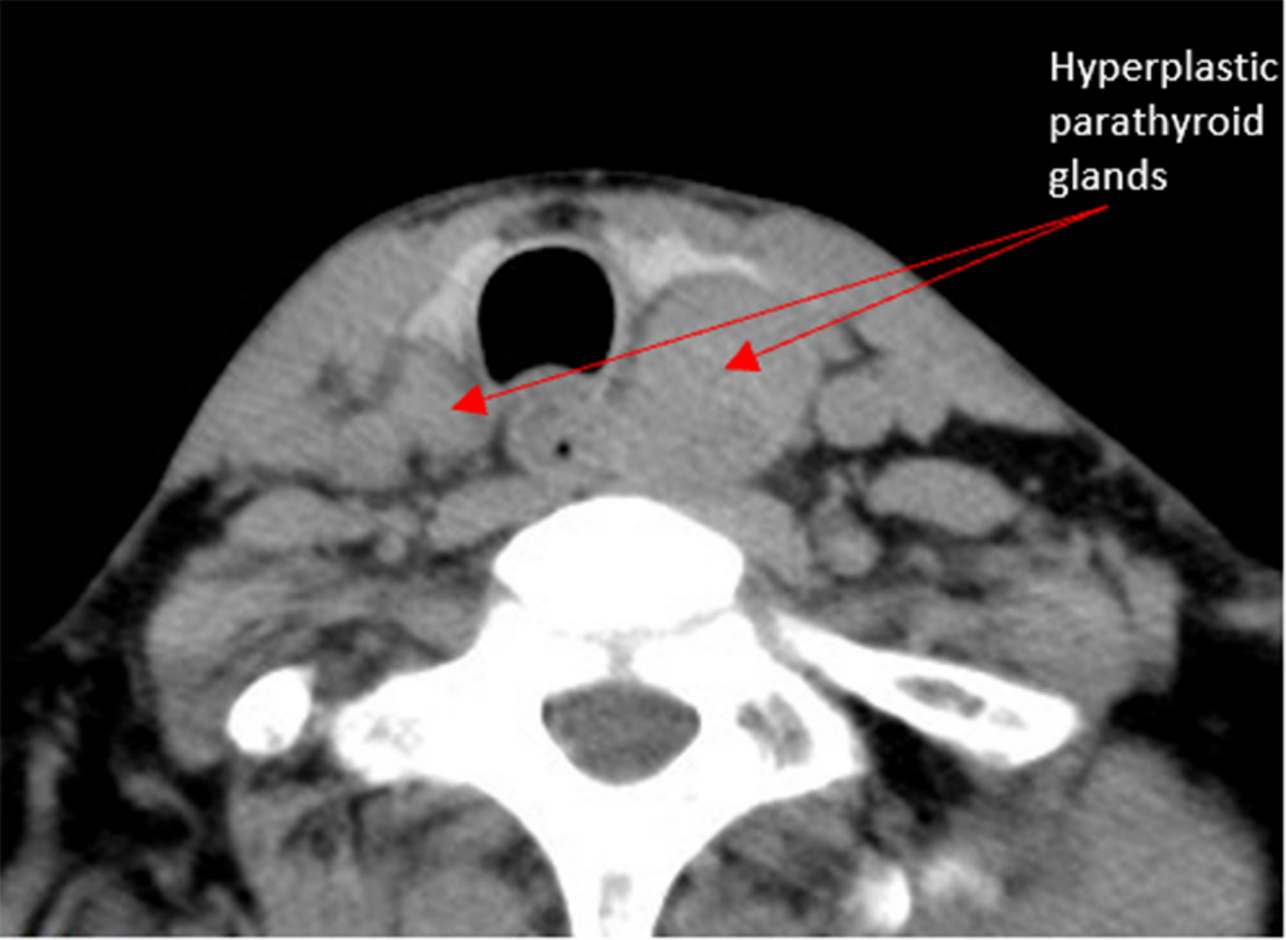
CT scan of the neck suggests parathyroid hyperplasia.
